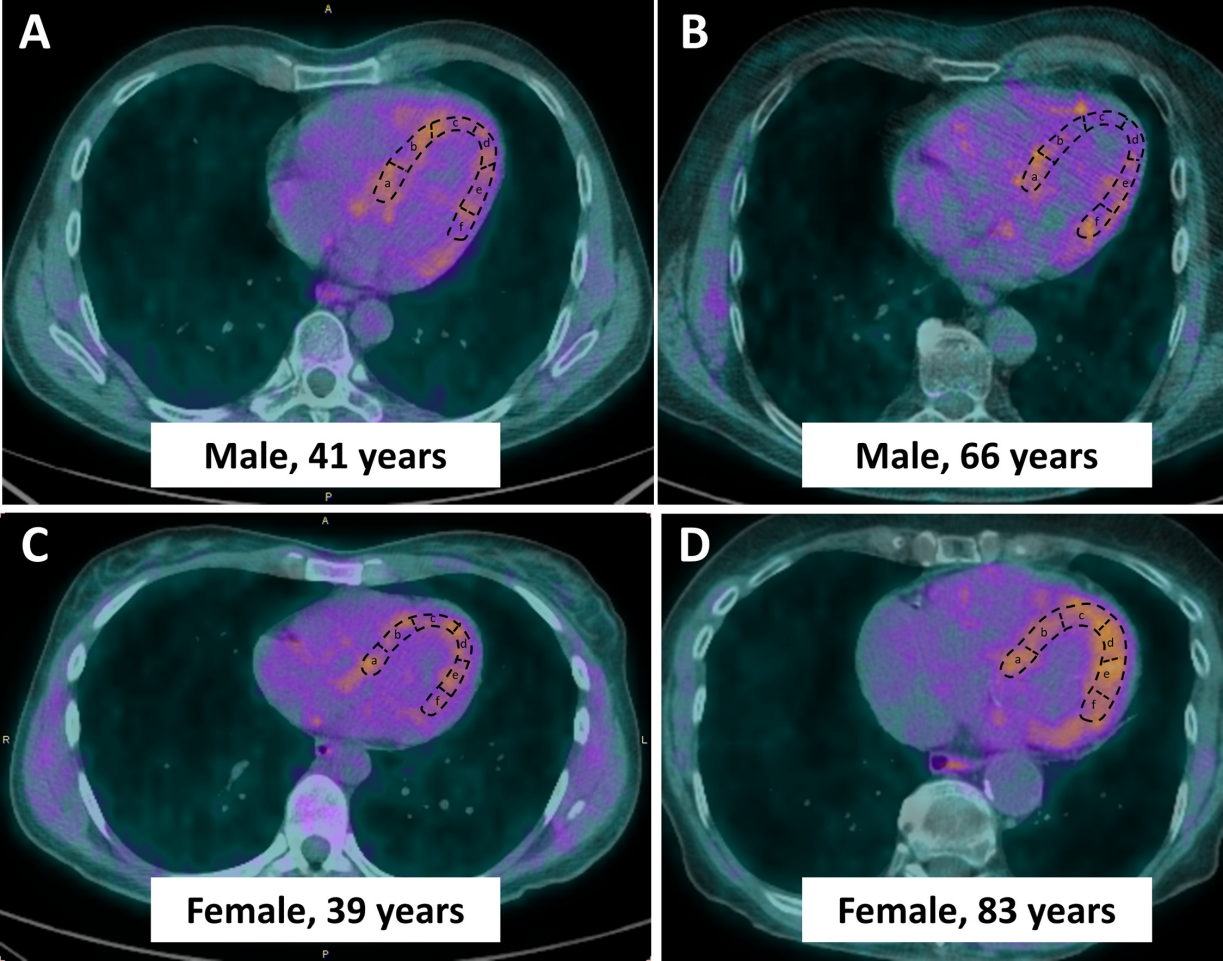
Example of Positron-Emission-Tomography image reconstruction using the pmod cardiac PET modelling tool (pmod version 3.8, PMOD technologies LLC, Zurich, Switzerland) and quantification of ^18^F-DOPA uptake in the left ventricular wall. A semiquantitative uptake analysis using the upper limit of the standardized uptake value (SUV_max-N_) and the mean SUV (SUV_mean-N_) at the sites of physiologic uptake was performed using a planar circular region of interest of 1 cm diameter, automatically generated by the computer. Left ventricular segments in the horizontal long axis were defined as follows: a = basal septal, b = midventricular septal, c = apical septal, d = apical lateral, e = midventricular lateral, f = basal lateral. A. Cardiac long axis image of a 41 year old male patient. B. Cardiac long axis image of a 66 year old male patient. C. Cardiac long axis image of a 39 year old female patient. D. Cardiac long axis image of a 72 year old female patient. Similar thresholds were applied for all images.
